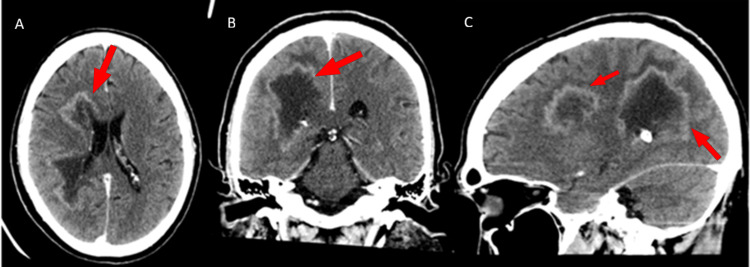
Postcontrast CT on presentation On postcontrast imaging, the lesion demonstrated peripheral enhancement in the involved regions as shown in the axial (A), coronal (B), and sagittal (C) planes. The enhancement pattern is highlighted by the red arrows.
